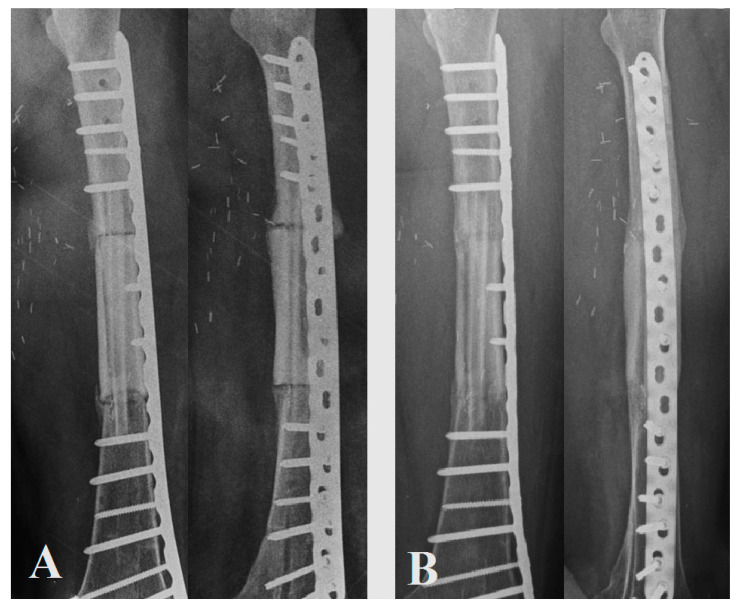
(A) Control X-rays for patient No. 1 at 3 months postoperatively; (B) control X-rays for patient No. 1 at 10 months postoperatively.
